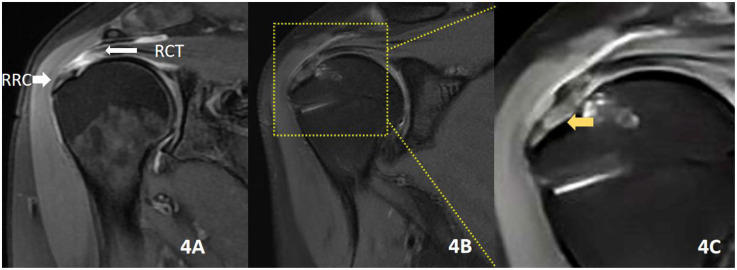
Male patient, 53 years old, with a traumatic injury causing shoulder pain and discomfort for 3 months, who underwent remnant-removal technique repair. (A) RCT (proximal rotator cuff tear), RRC (remnant rotator cuff in the footprint area). (B) MRI of the shoulder joint repeated 2 years after surgery. (C) Shows B 3× magnified image in the yellow box; the yellow arrow indicates the repair after the RR technique. The tendon–bone healing interface is scar healing without the original tissue of the rotator cuff migration area.
